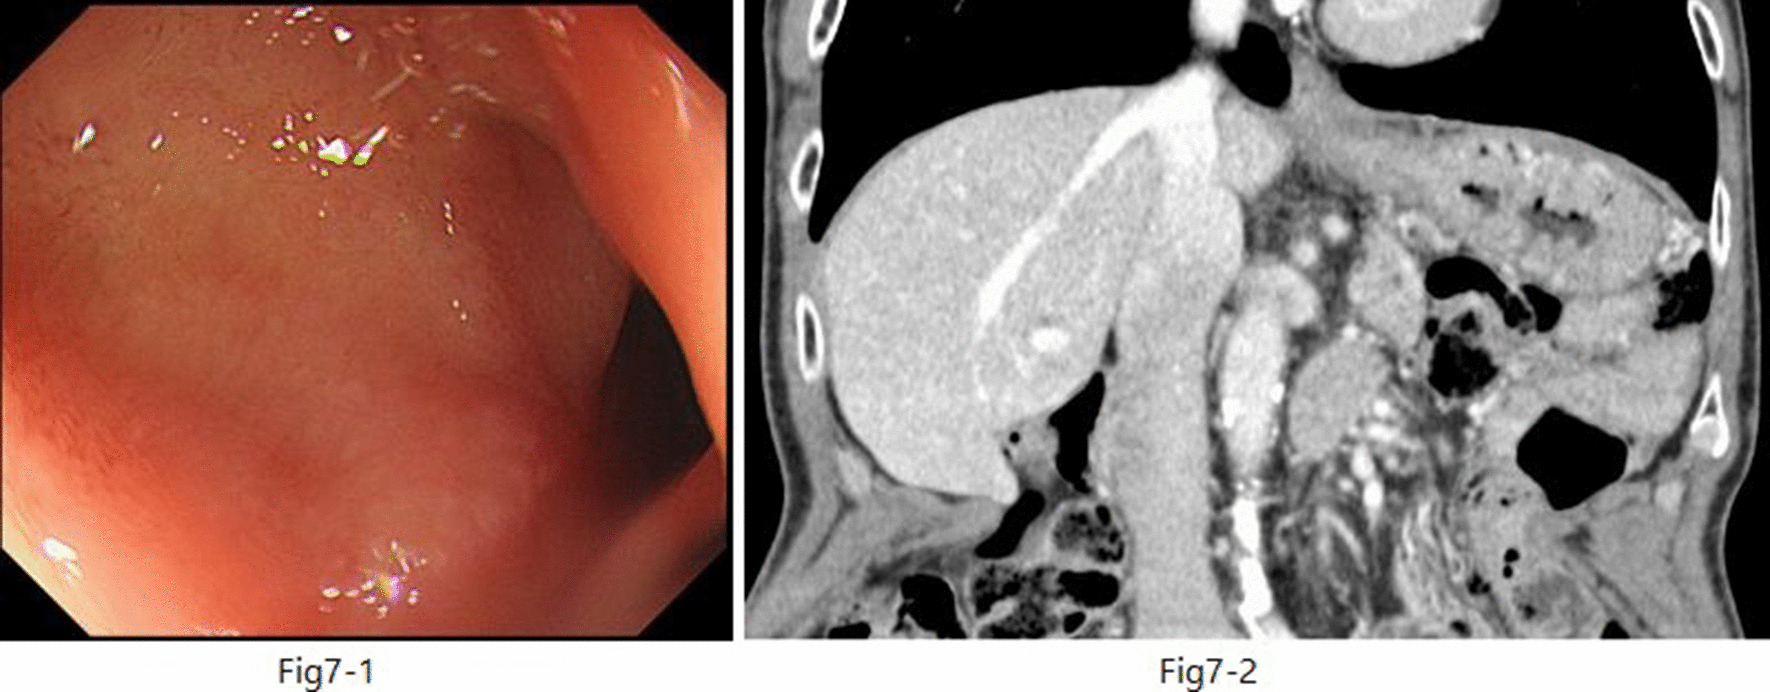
(1) Gastrointestinal fiber copy; the ulcer has healed. (2) Computed tomography scan (coronal view) showing that the abscess has disappeared and the tube has also been removed
